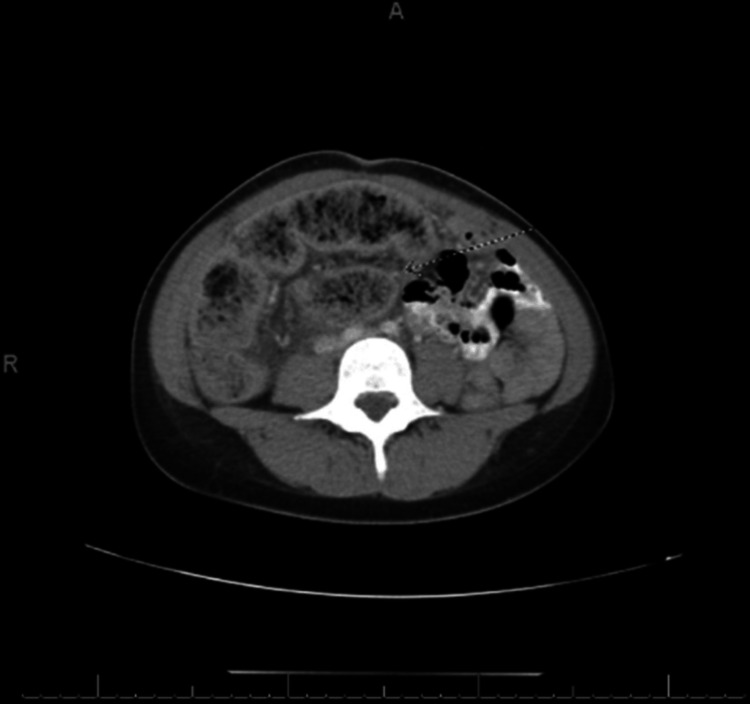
CT abdomen and pelvis demonstrating a cecal volvulus (arrow). CT, computed tomography
